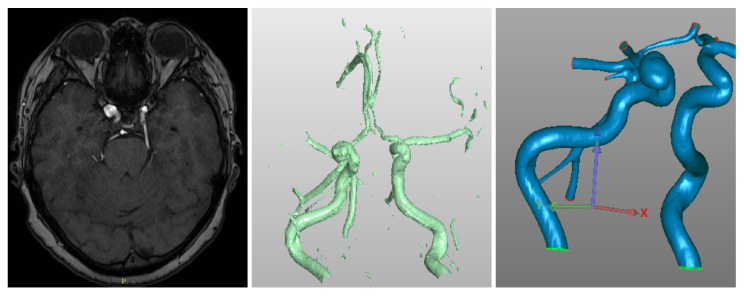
Overview of reconstruction process, from left to right: (left) a 3D model of vessels is built from a 3D CT-angio DICOM images; (center) Preparing continuos STL model via ITK Snap + 3D Viewer software; (right) finalization of the model via SolidWorks software (Boston, MA, USA).
